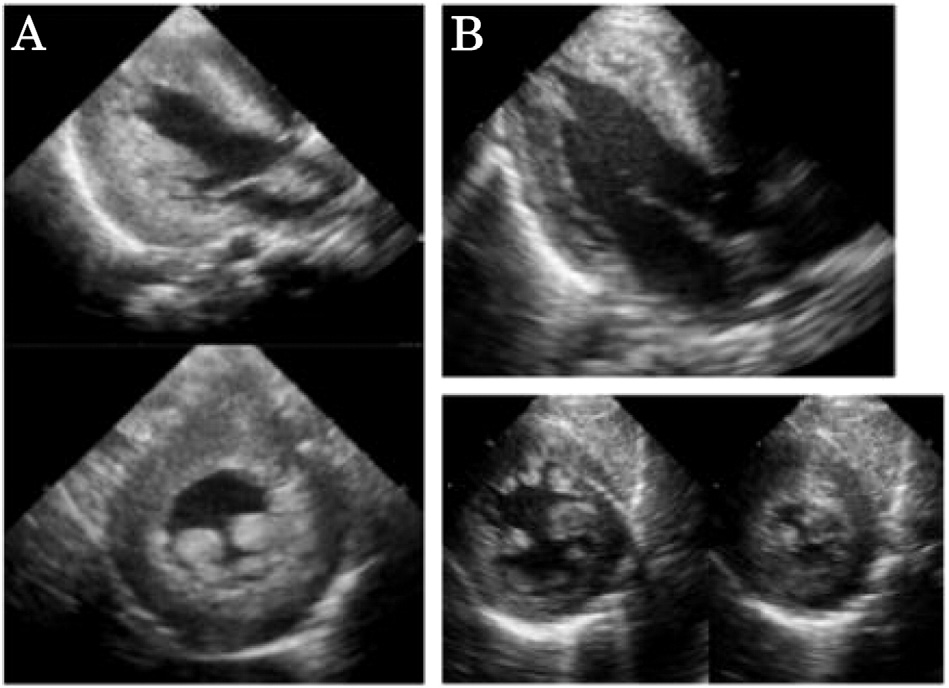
Echocardiography (ECHO) findings before ERT in two siblings. A. Patient 1 at 4 months of age and before ERT. ECHO shows remarkable left ventricular wall thickening with a left ventricular end-diastolic posterior wall thickness (PWd) of 14 mm (328% of normal) and an inter-ventricular septum end-diastolic thickness (IVSd) of 12 mm (307% of normal). The left ventricular end-diastolic dimension (LVIDd) was 25.8 mm (98% of normal), and there was no left ventricular outflow stenosis. B. Patient 2 at birth and before ERT. LVMI was 120 g/m^2^ (198% of normal), which was calculated by ECHO.
